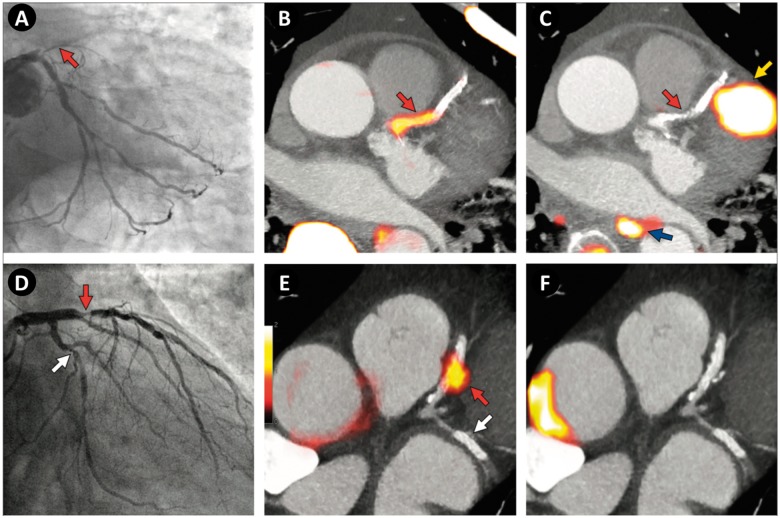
Focal ^18^F-fluoride and ^18^F-fluorodeoxyglucose uptake in patients with myocardial infarction and stable angina. (Top row, *A*–*C*) Patient with acute ST-segment elevation myocardial infarction with (*A*) proximal occlusion (red arrow) of the left anterior descending artery on invasive coronary angiography and (*B*) intense focal ^18^F-fluoride uptake (yellow-red) at the site of the culprit plaque (red arrow) on the combined positron emission and computed tomography coronary angiography (PET-CTCA). Corresponding ^18^F-fluorodeoxyglucose PET-CT image (*C*) showing no uptake at the site of the culprit plaque. Note the significant myocardial uptake overlapping with the coronary artery (yellow arrow) and uptake within the oesophagus (blue arrow). (Bottom row) Patient with anterior non-ST-segment elevation myocardial infarction with (*D*) culprit (red arrow; left anterior descending artery) and bystander non-culprit (white arrow; circumflex artery) lesions on invasive coronary angiography that were both stented during the index admission. Only the culprit lesion had increased ^18^F-NaF uptake on PET-CT (*E*) after percutaneous coronary intervention. Corresponding ^18^F-fluorodeoxyglucose PET-CT (*F*) showing no uptake either at the culprit or the bystander stented lesion. Note intense uptake within the ascending aorta. Adapted from Joshi *et al.*^47^
